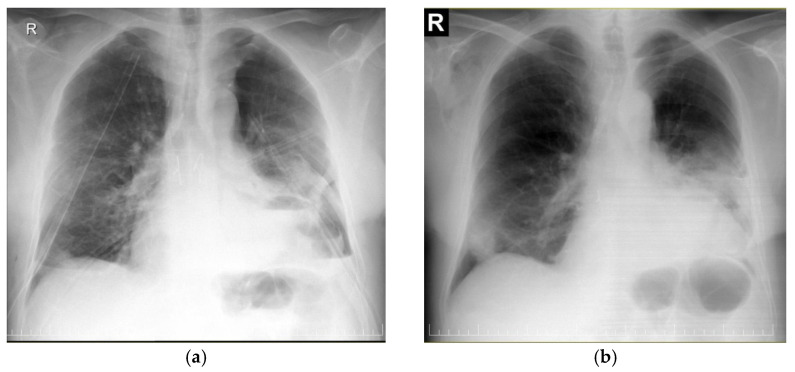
Conventional chest radiography on postoperative day 4 with bilateral pleural drainage, and small left basal pneumothorax still present, as the left lung initially could not re-expand fully (a), and at discharge with closed left pleural space by smaller left lung and elevated left hemidiaphragm (b).
